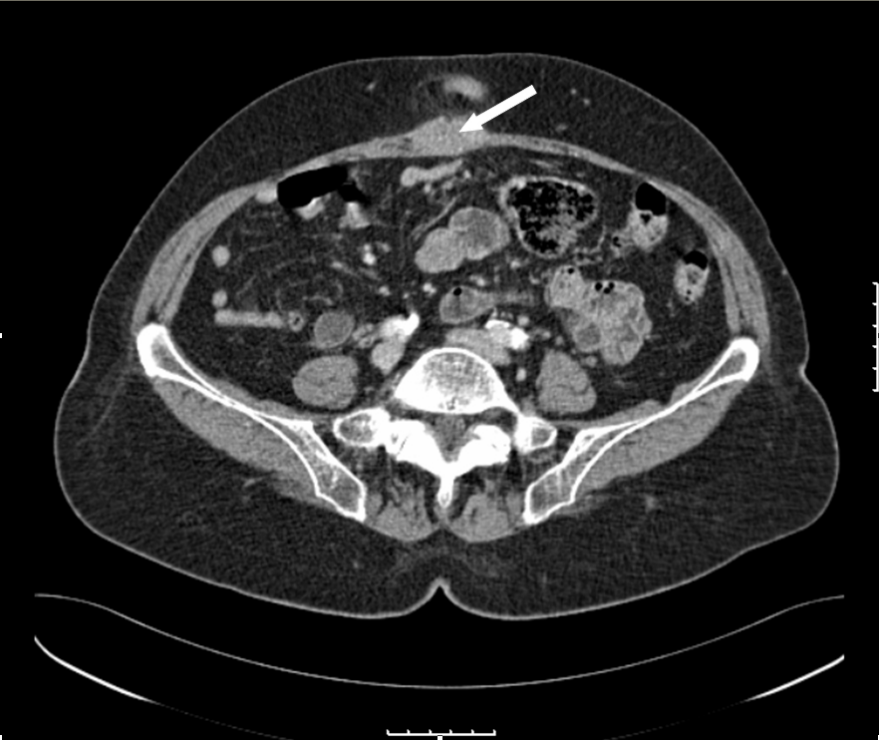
CT scan of abdomen showing soft tissue mass in the anterior abdominal wall (white arrow). The ventral incisional hernia is seen on this slice and was arising cranially but lies superiorly to the mass.
